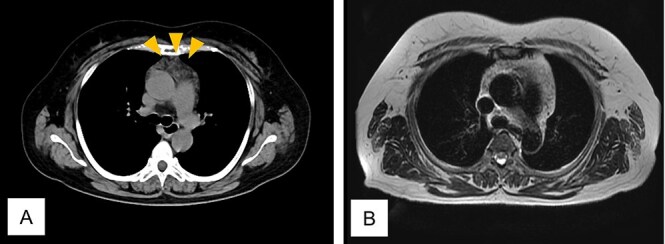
Computed tomogram and magnetic resonance image of Case 2. (A) Chest computed tomogram shows a 4 x 3 cm anterior mediastinal mass with increased fat attenuation (arrowhead). (B) T2-weighted magnetic resonance image of the chest shows heterogeneous fatty signal within the thymus, but no solid component is observed.
